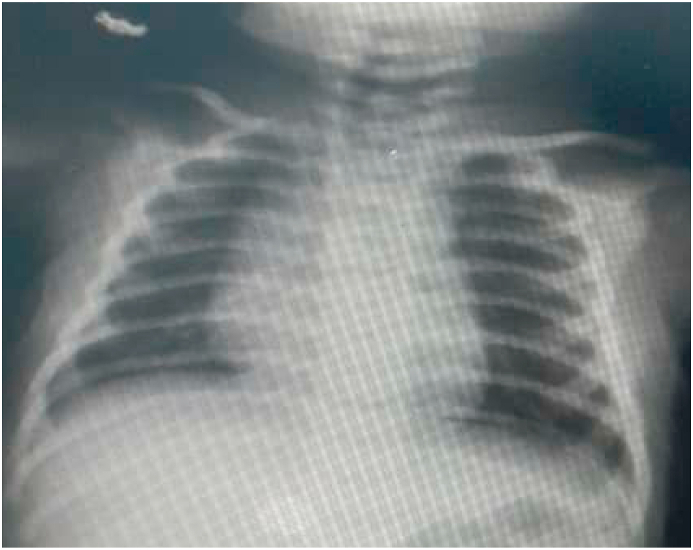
Follow-up X-ray after 20 days of operation shows normal findings.
